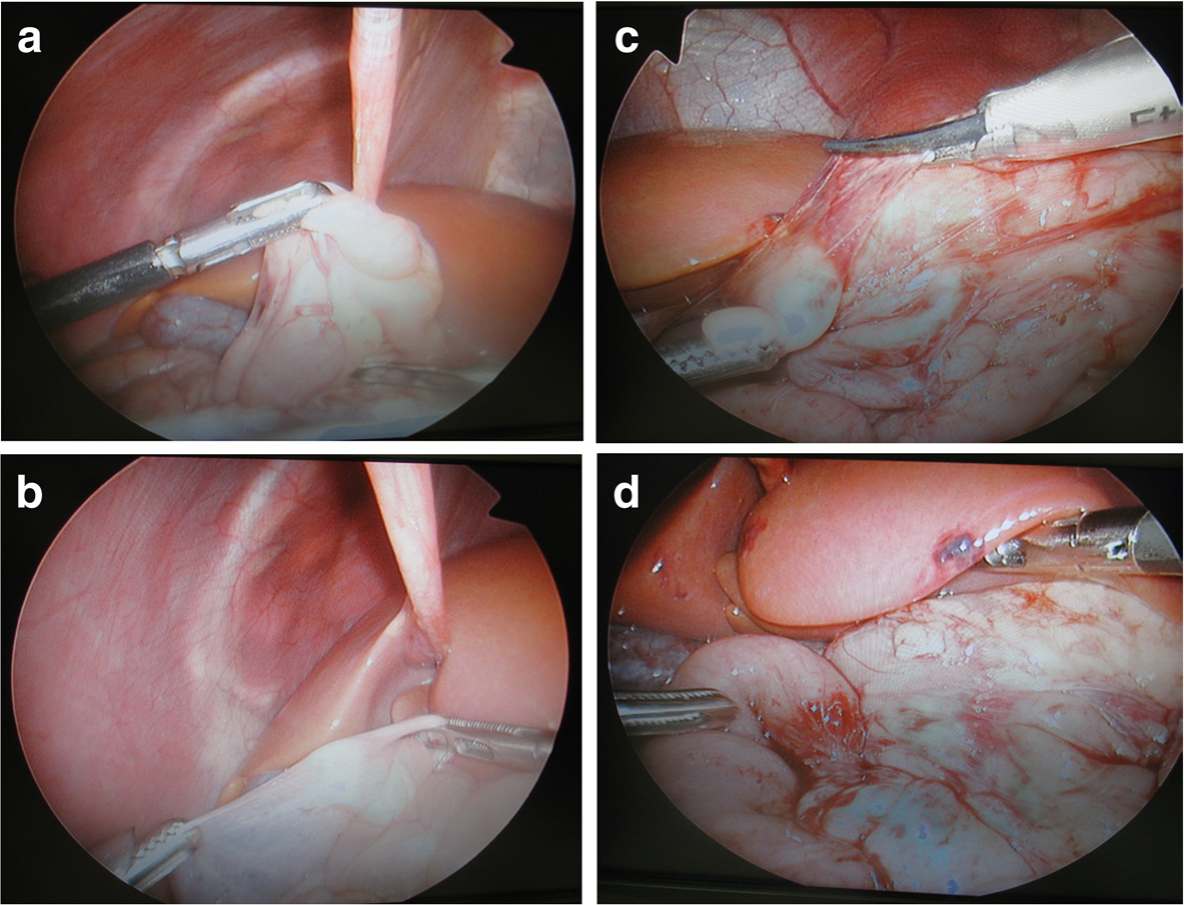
Operative view. a The cecum was located just under the liver at mid-abdomen. b, c The thick Ladd’s band was dissected using ultrasonic surgical device. d Mesentery was widened with dissection of interlaced thin ligaments on the anterior surface of mesentery
